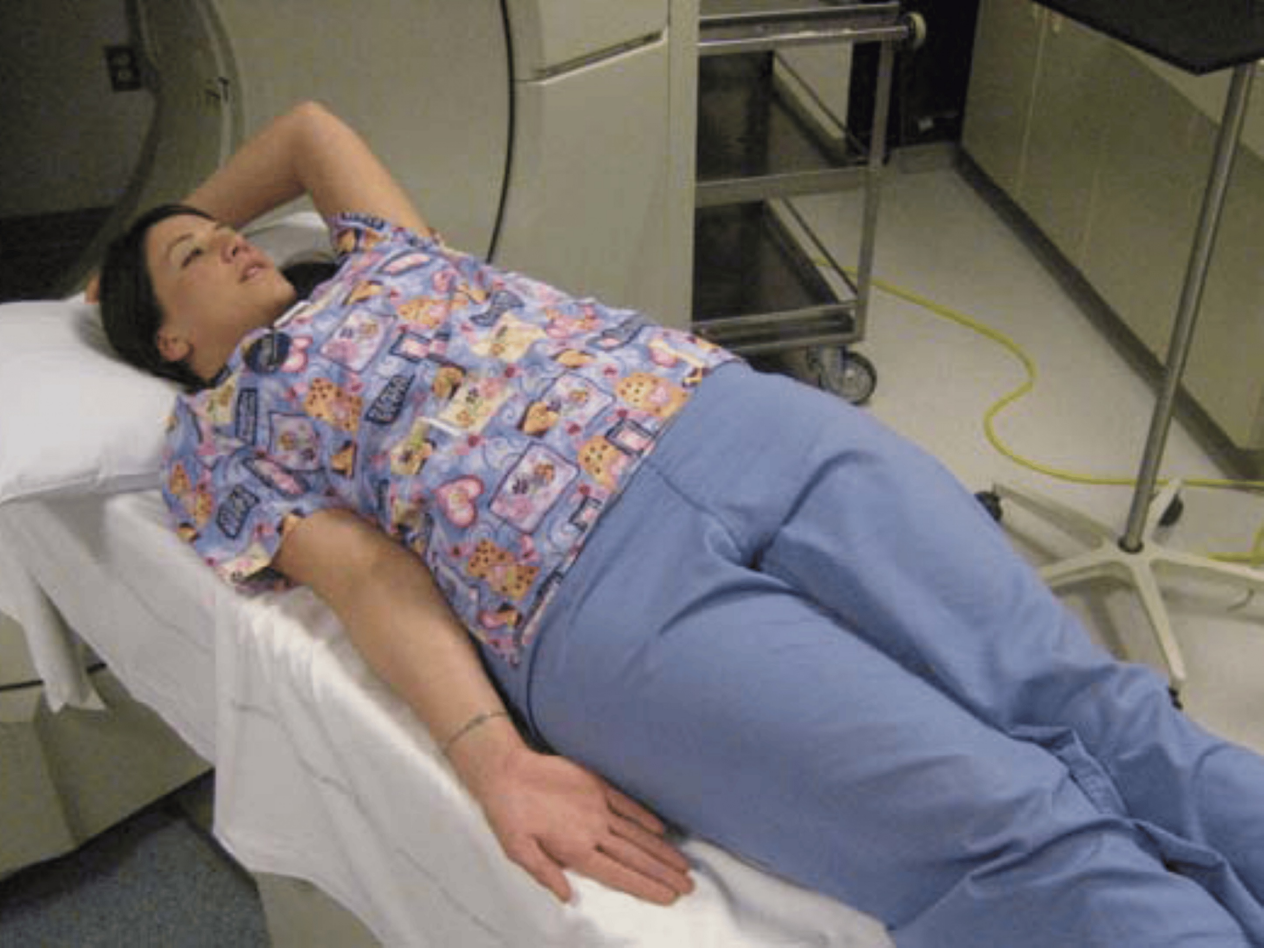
Patient position for MRI shoulder Adapted from AHI MSK CT Protocols (https://xanatomy.net/CTPImages/Shoulder-position.png)
